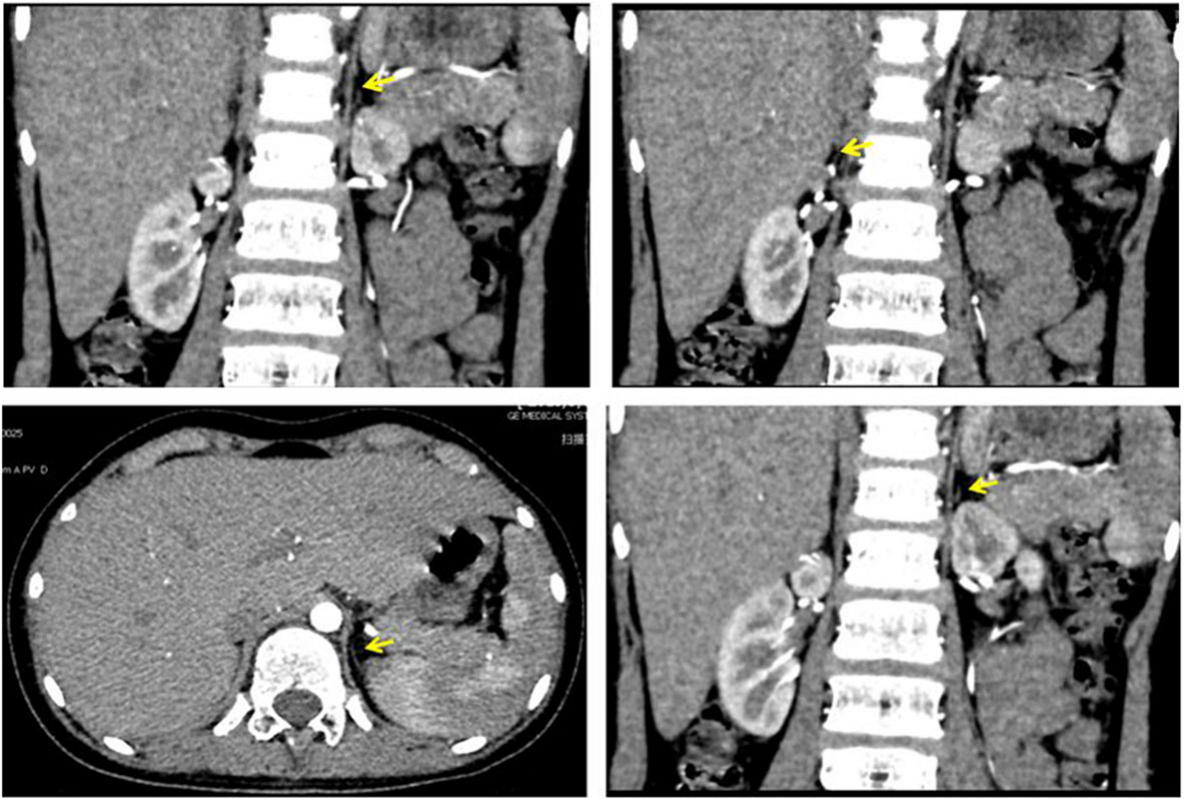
At the age of 13.2-year adrenal computed tomography (CT) scans revealed that the adrenal glands on both sides were much smaller than normal. The yellow arrows indicate the adrenal gland
